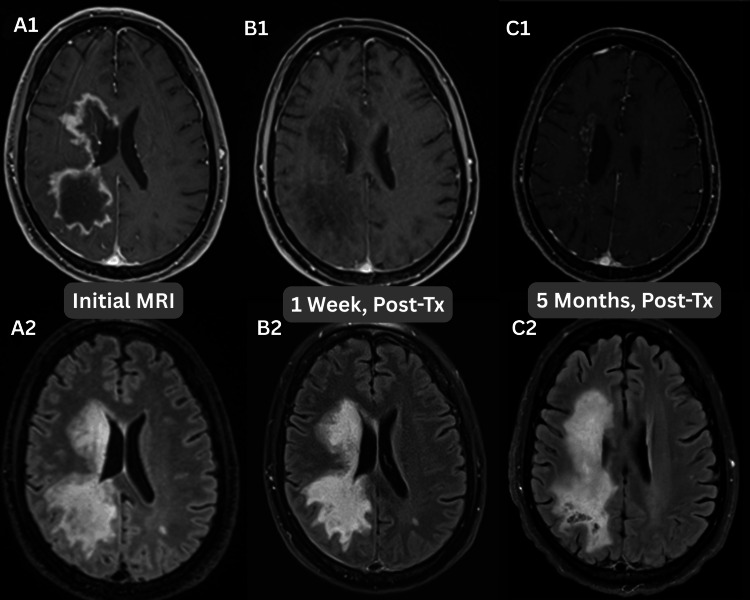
MRI post-contrast and T2 FLAIR Axial slice images at the level of the lesion at different time points, presenting on both T1-weighted and T2 fluid-attenuated inversion recovery (FLAIR) sequences. Images A1 and A2 are from the initial presentation, B1 and B2 are from one week post-treatment, and C1 and C2 are from five months post-treatment.
